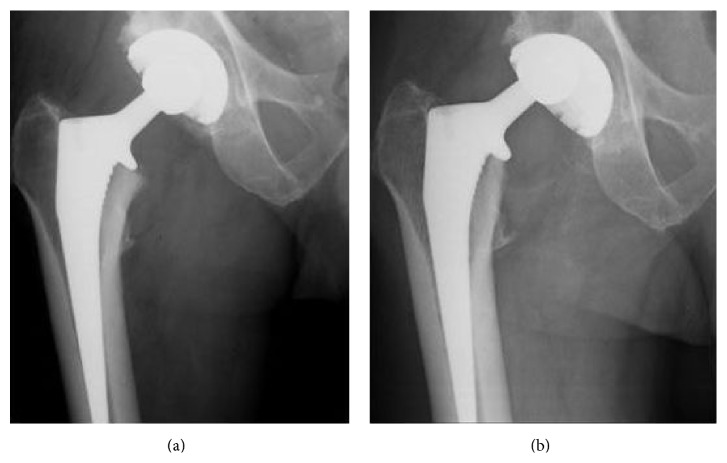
(a) Previous AP Hip X-ray demonstrates satisfactory component positioning. (b) AP Hip X-ray from ED attendance demonstrates acetabular liner dissociation. Comparison to (a) shows grossly eccentric superior migration of the femoral head within the acetabular shell.
